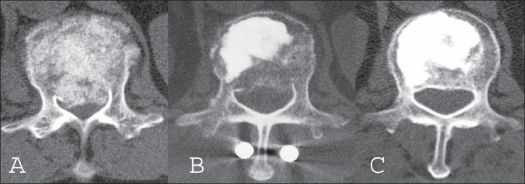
Axial CT scan (A) image shows posterior cortical bone retropulsion. and spinal canal narrowing of 67%. Postoperative Axial CT scan (B) following surgery demonstrating adequate HA graft placement and reduction of the retropulsed bony fragment. Axial CT scan (C) of 2 years after surgery demonstrating further improvement of the spinal canal narrowing and radiographic healing of the vertebral body
